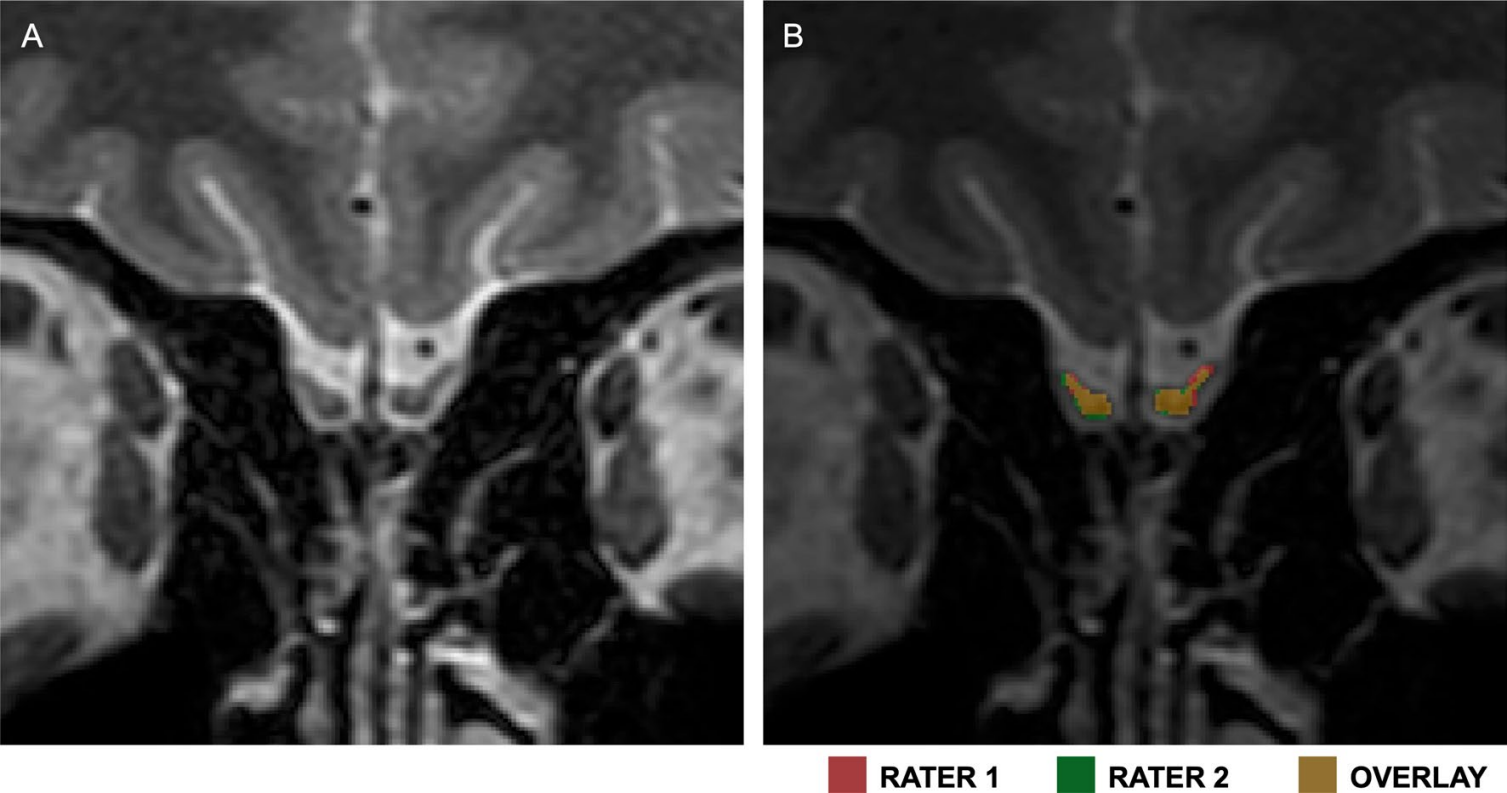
Manual segmentation of the olfactory bulbs. A. Coronal T2-weighted image of a participant. B. Coronal T2-weighted image with segmentations made by the two evaluators. Red: Rater 1; Green: Rater 2; Yellow: Overlap.
